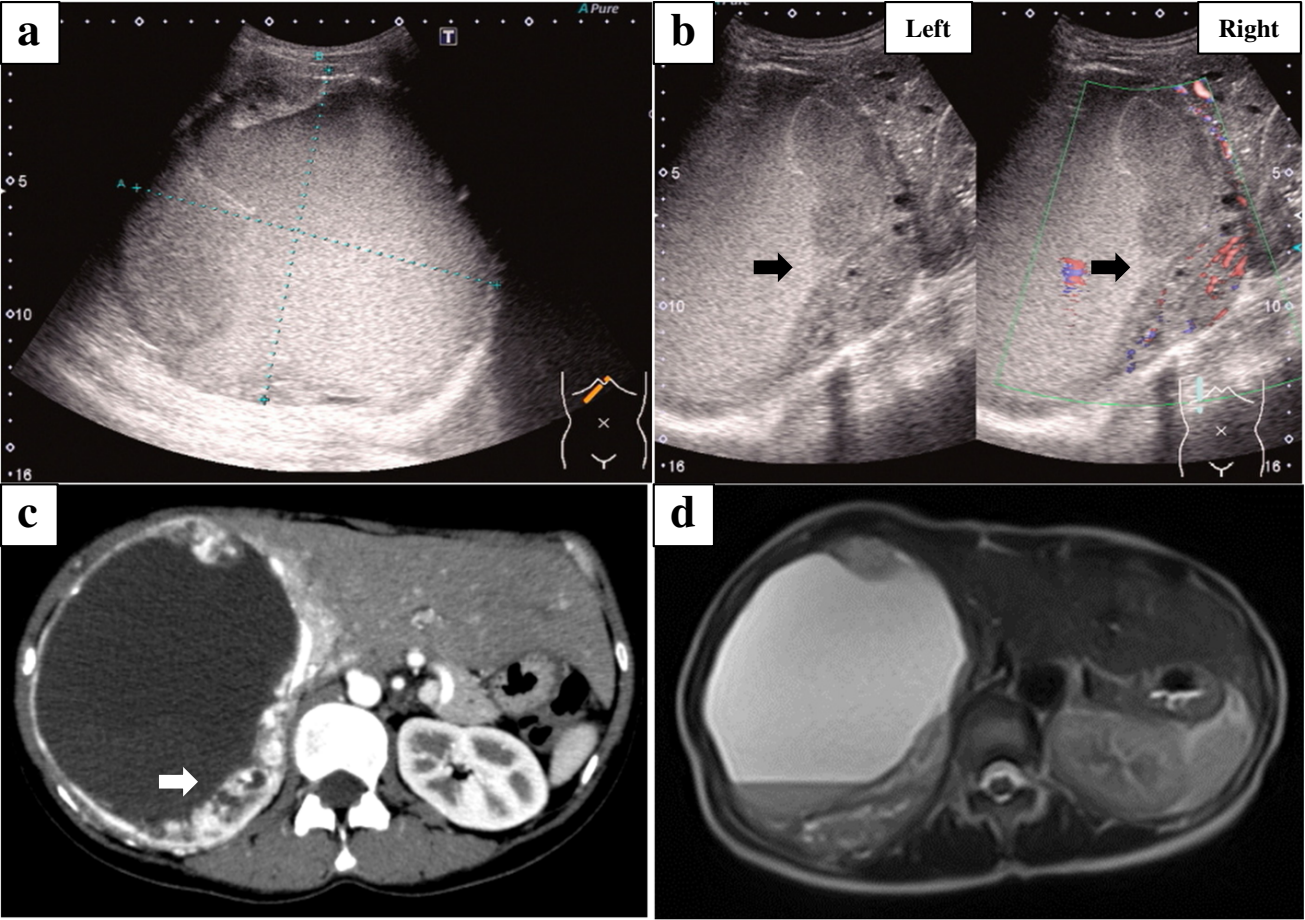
Findings from abdominal ultrasonography, CT, and MRI. a Abdominal ultrasonography shows an extensive space-occupying lesion in the right lobe of the liver, 15 cm in diameter. b
